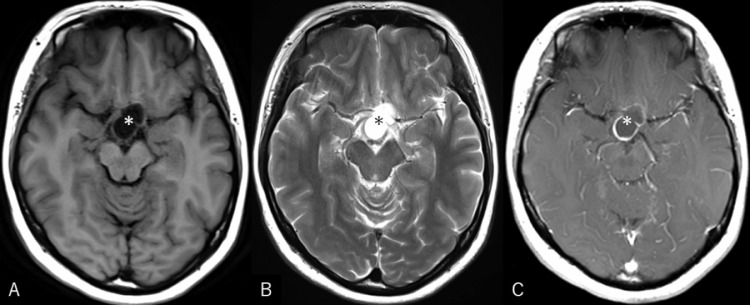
Axial T1- (A), T2- (B), and postcontrast T1-weighted (C) magnetic resonance images demonstrating the cyst, 25 mm × 15 mm in maximal dimension, which exhibits low intensity on T1- and high intensity on T2-weighted imaging with marginal enhancement (A-C, asterisk).
